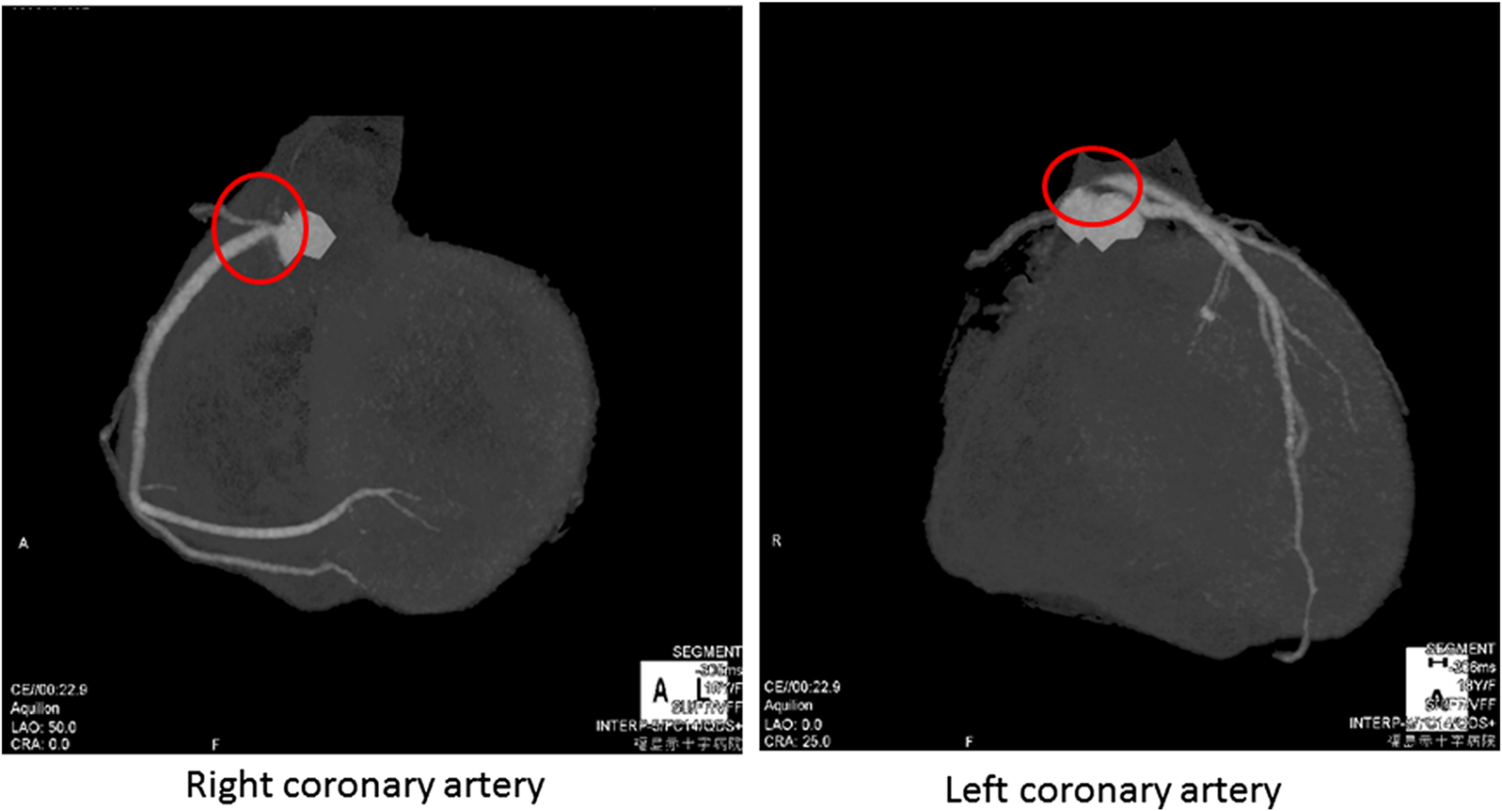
Coronary CT angiography. Severe stenoses were observed in the ostium of the left main trunk and the right coronary artery
